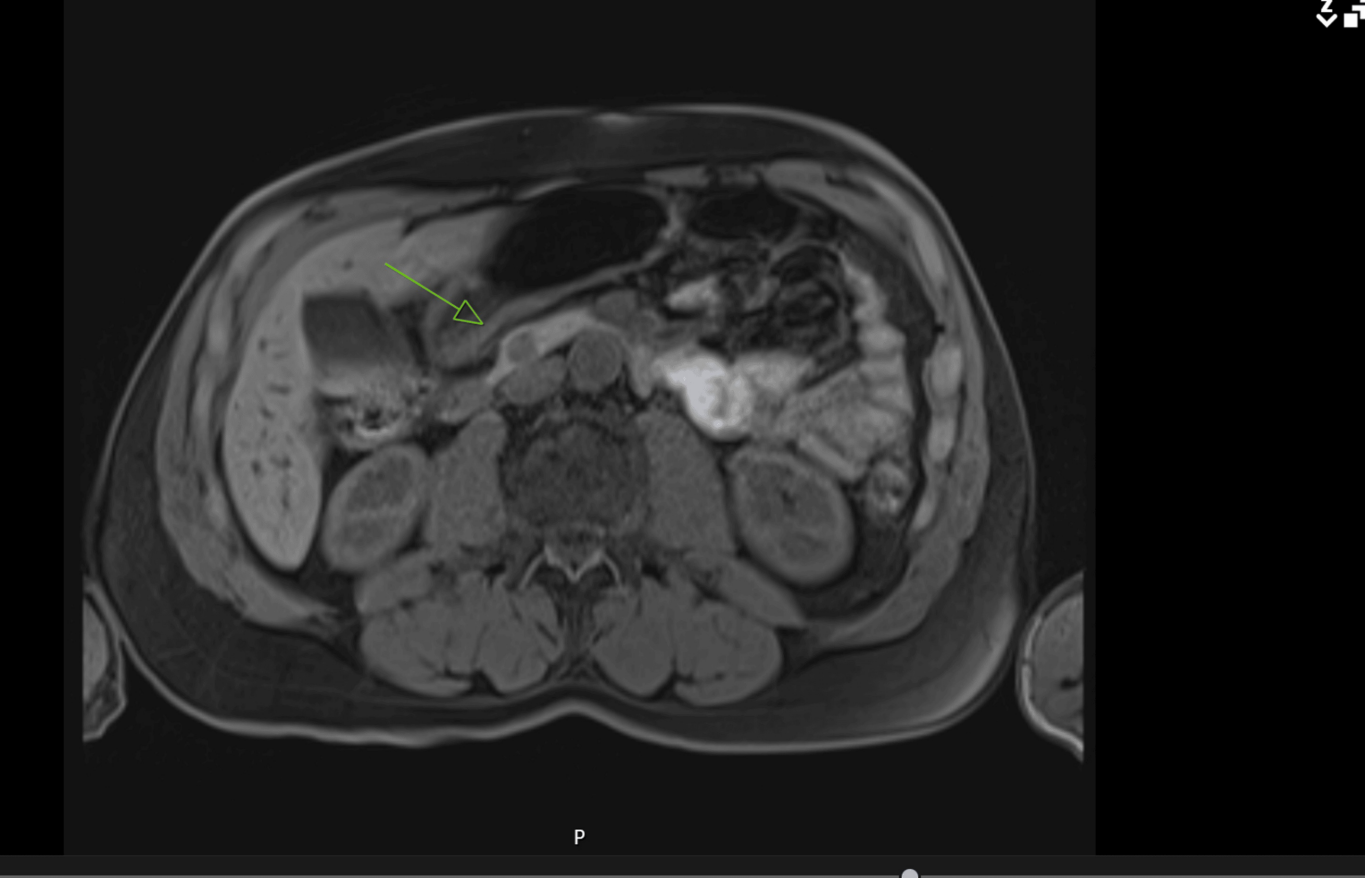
MRI of the pancreas showing a 10 mm nodule located within the inferior pancreatic head, just below the level of the ductal insertion at the ampulla
